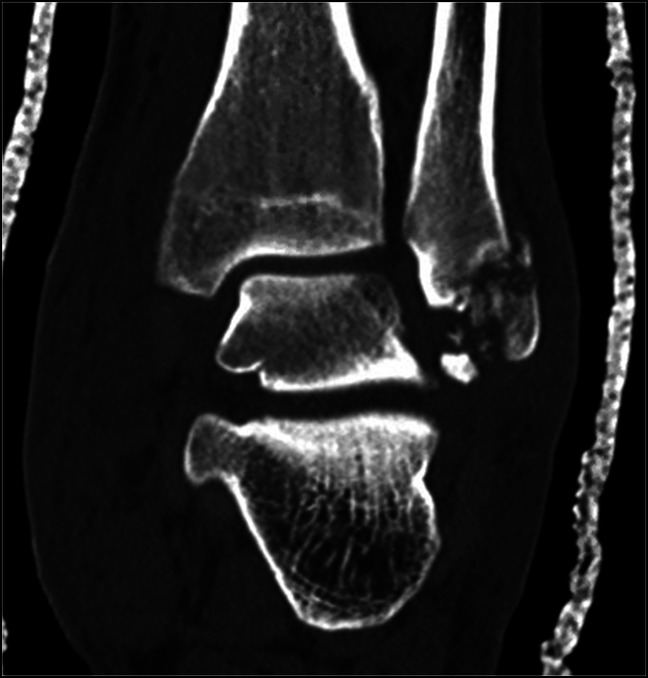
Immediate postreduction coronal CT image showing realignment of the ankle and subtalar joints with fracturing of the posterolateral fibula.
